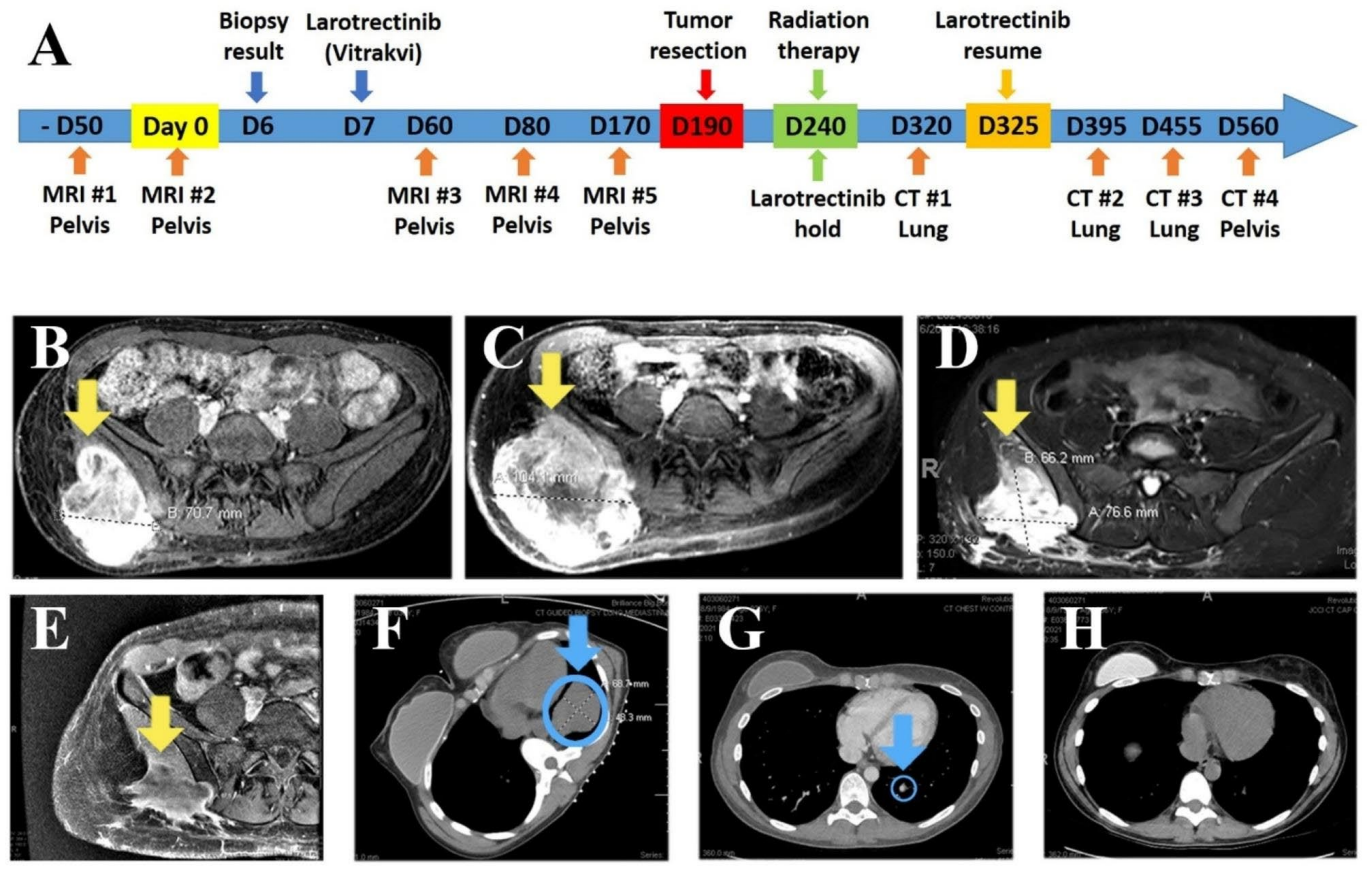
Clinical timeline (A) and imaging (B-E). Before treatment (B: - Day 50 & C: Day 0), a heterogenous mass (yellow arrows in B-E) centers in the right gluteus medius and maximus muscles with extension into the right paraspinal musculature, and invasion of the right posterior iliac bone. Imaging on post-treatment Day 60 (D) and Day 170 (E) show significant decrease in tumor size (MRI#4 of Day 80 is not shown here). 130 days after surgery, a 6.9 cm solid mass in the lower lobe of left lung is biopsied and proven to be a metastatic lesion (F, Day 320, blue arrow with circle), with near complete resolution after the resumption of Larotretinib treatment (G: Day 455, blue arrow with circle & H: Day 560)
